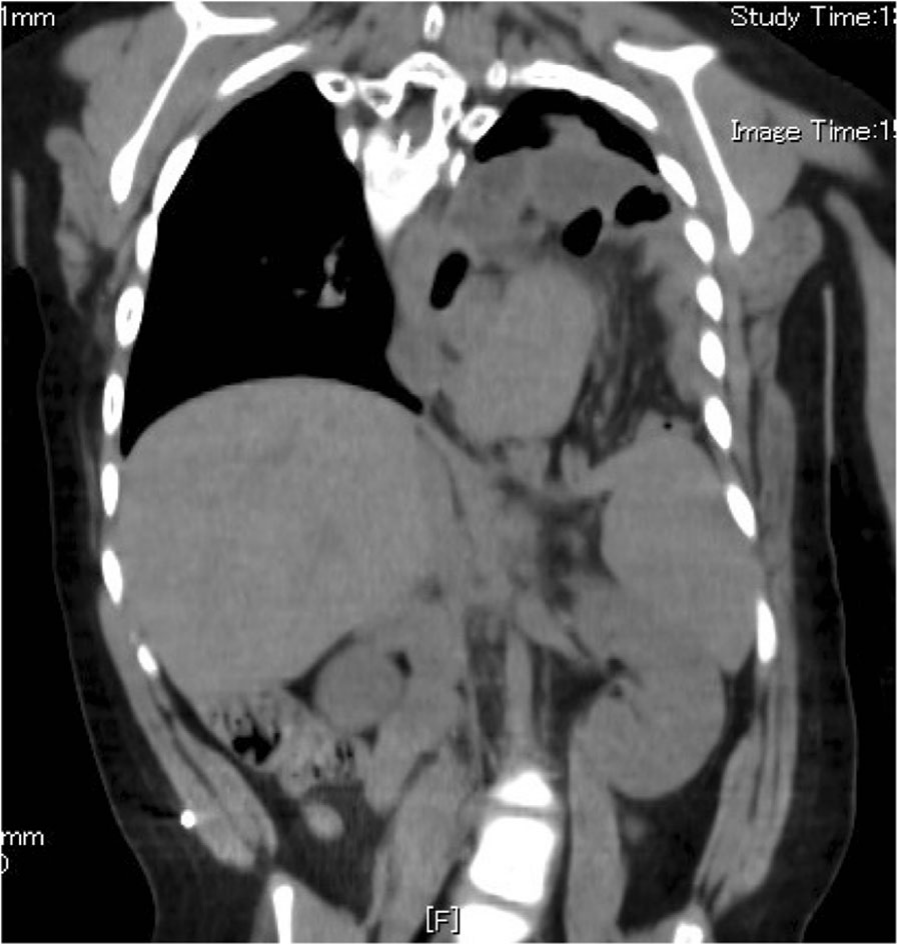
A CT scan revealed the herniation of the stomach and the large and small intestine into the thoracic cavity
